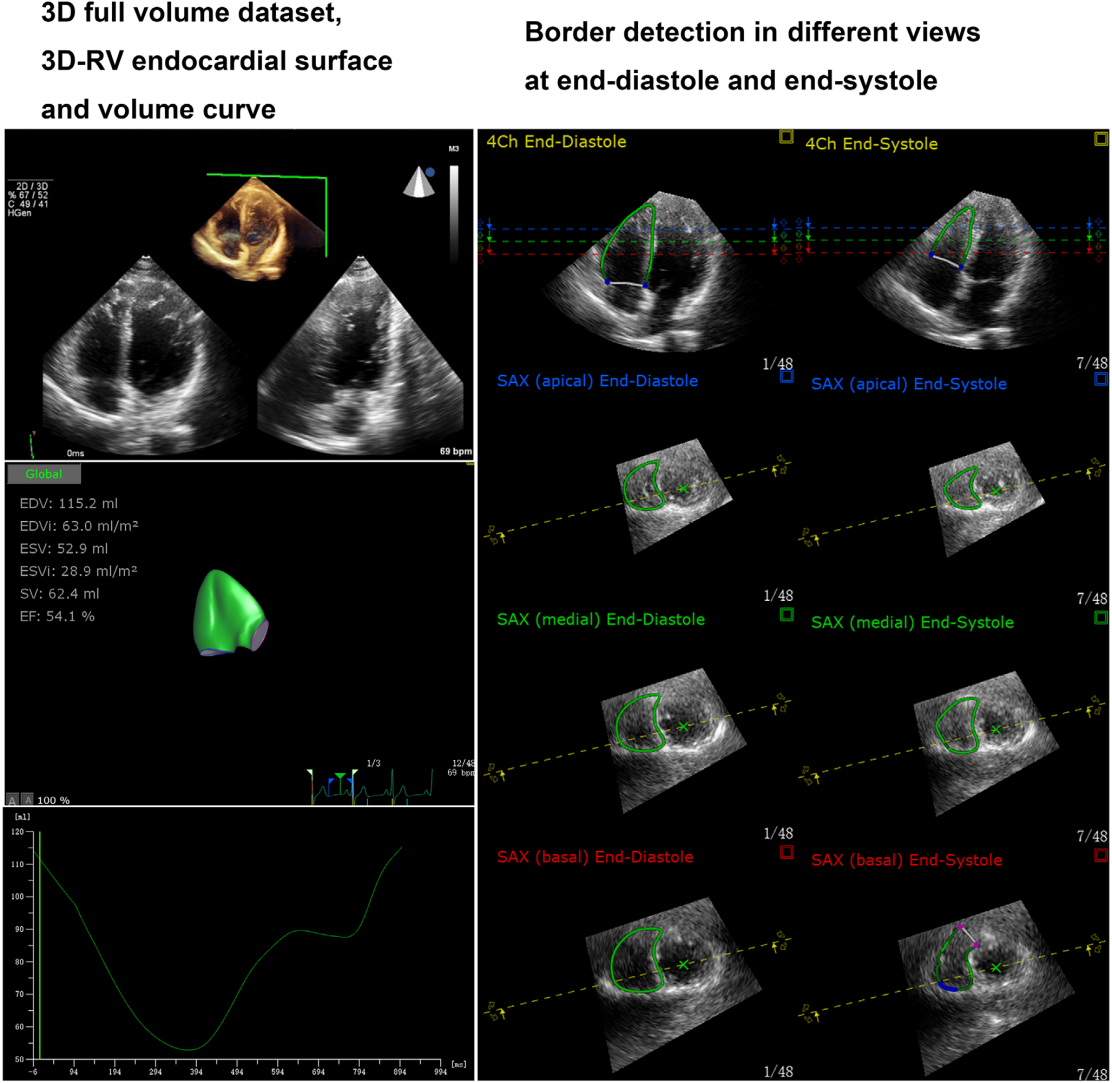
Three‐dimensional‐right ventricular (3D‐RV) image analysis was performed with the vendor‐independent software Tomtec. Left panel: 3D images of the RV‐focused apical four‐chamber view, the reconstructed 3D‐RV model and the RV volume‒time curve are displayed. Right panel: different slices of the 3D‐RV model at end‐diastole and end‐systole are shown.
